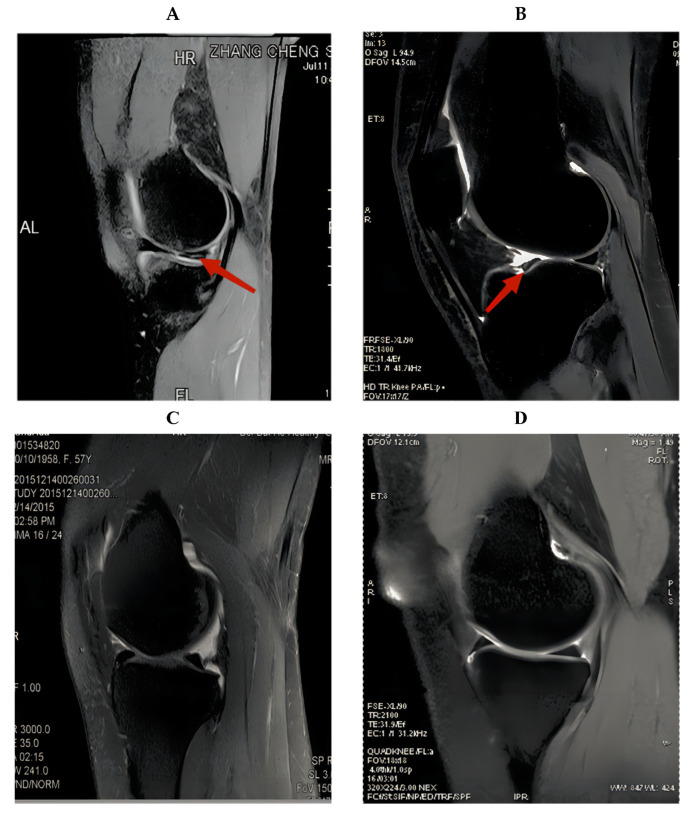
MRI images of knee joint with meniscus injury. (A) The posterior root injury of the meniscus. (B) The anterior root injury of the meniscus. (C,D) MRI images of knee joint without meniscus injury. The arrows point to the meniscus injury.
